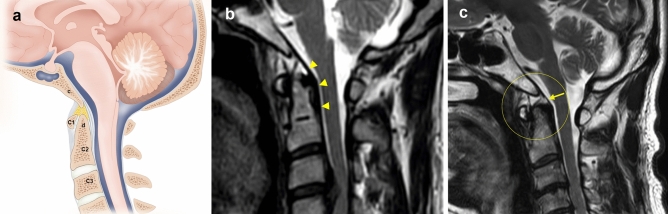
Sagittal view of the craniovertebral junction. (a) The cruciate ligament–tectorial membrane (TM)–dura mater complex between the clivus and the body of the axis. *c* clivus, *d* dens. (b) Sagittal T2-weighted magnetic resonance image shows thin normal delineated TM with normal integrity from clivus to dens (arrowheads). (c) Sagittal T2-weighted magnetic resonance image shows a discontinuous TM (asterisks) surrounded by fluid signal intensity. In a patient with TM tear, the TM shows attachment to the superior clivus. The TM tear is visualized at the level of apex of the dens (arrow). At the level of the body of the axis, the TM shows irregular fraying, and the continued posterior longitudinal ligament is retracted with coarse attachment to the dens.
